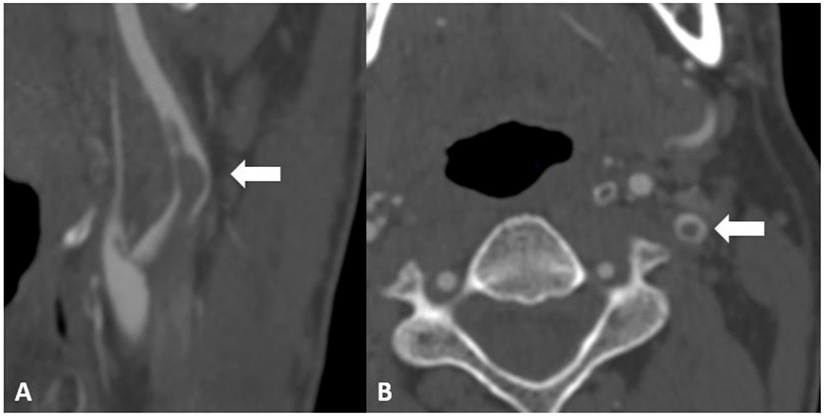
Computed tomography angiography image of a free-floating thrombus in the proximal left internal carotid artery. (A) depicts a sagittal view of the carotid bifurcation with a contrast filling defect corresponding to a free floating thrombus extending into the distal internal carotid artery lumen (arrow). (B) depicts an axial view of the internal carotid artery with a central contrast filling defect corresponding to the free-floating thrombus in the internal carotid artery.
